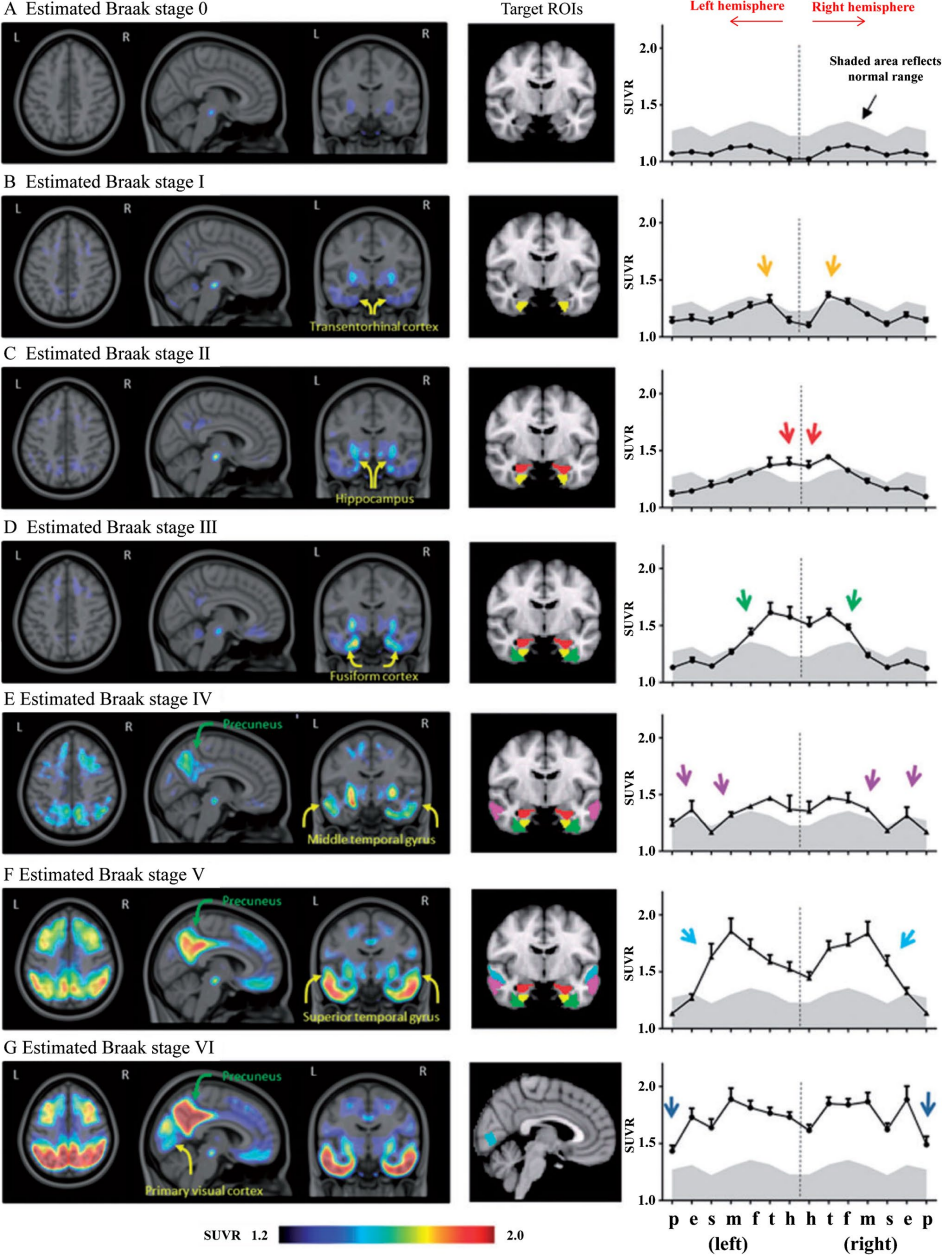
Average SUVR images of.^18^F-AV-1451 PET scans and region of interest profiles by estimated Braak stage. The regions of interest profiles are as showed “butterfly plots,” with the regions of interest from each hemisphere mirrored and ordered mediolaterally, reflecting both the anatomical distribution and order of involvement consistent with disease progressiveness. (This research was originally published in Brain. 2016;139:1539–50. Reprint permission was obtained)
